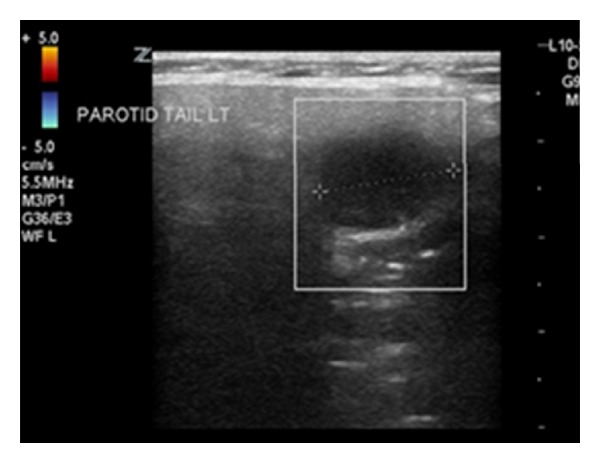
Ultrasound of parotid/neck region. Within the right parotid a 15 mm hypoechoic lesion was seen. Initial differentials: salivary gland tumour and abnormal possibly necrotic intraparotid node.
